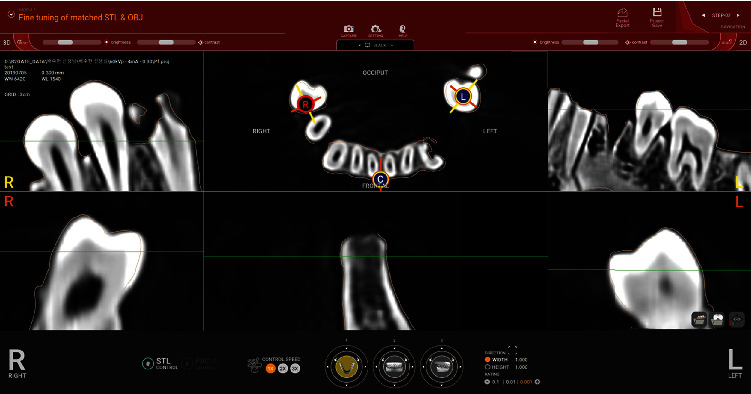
Image matching between cone-beam computed tomography and optical dental scan.
